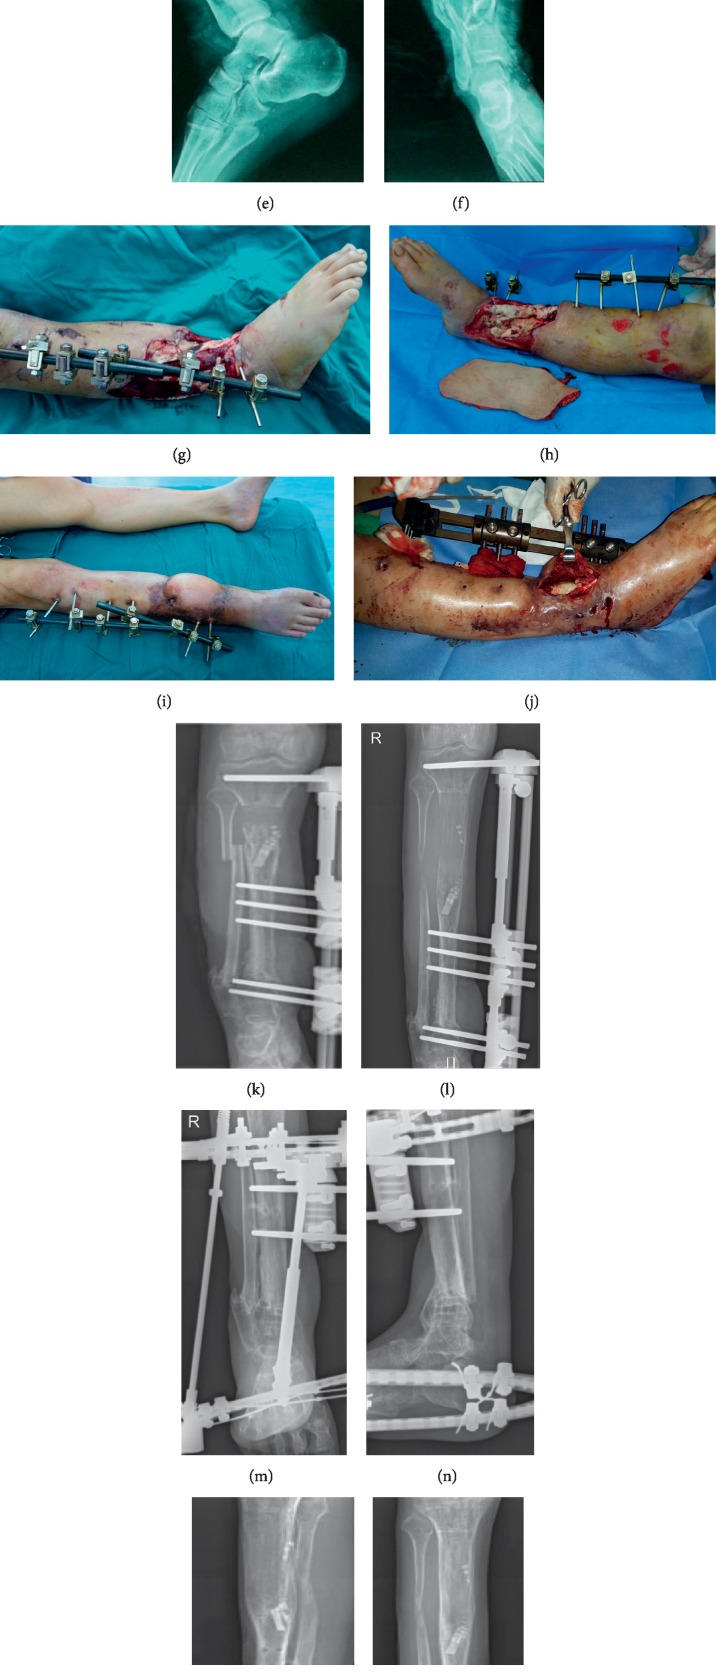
(a–f) Both the appearance and the X-rays of the injured lower leg; (g-h) free anterolateral thigh flap for repairing the wounds after multiple debridements and wound purification; (i-j) external fixator was adjusted, the lesions were debrided again, and osteotomy and bone transport were performed at the proximal tibia intraoperatively; (k-l) the X-rays of tibia and fibula during bone transport at approximately 2 months and 9 months. (m-n) Foot ring was added to correct foot drop in later stage of bone transport. 20 months after bone transport, the external fixator was scheduled to be removed. (o–r) Both the appearance and the X-ray at 36-month follow-up after the bone transport.
